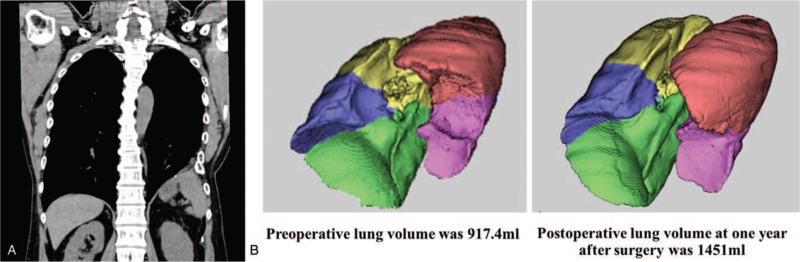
Postoperative chest X-ray and lung volumetry. (A) No recurrence of congenital diaphragmatic hernia on postoperative chest X-ray. (B) The volume of the compressed lung re-expanded from 917 mL to 1451 mL on lung volumetry.
